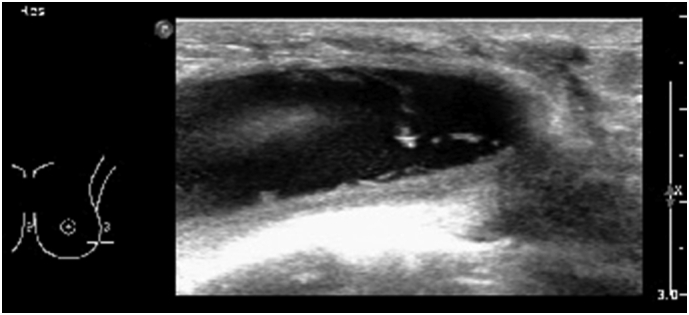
Left breast ultrasound depicting a thick wall of left breast with moderate amount of peri-implant fluid.
